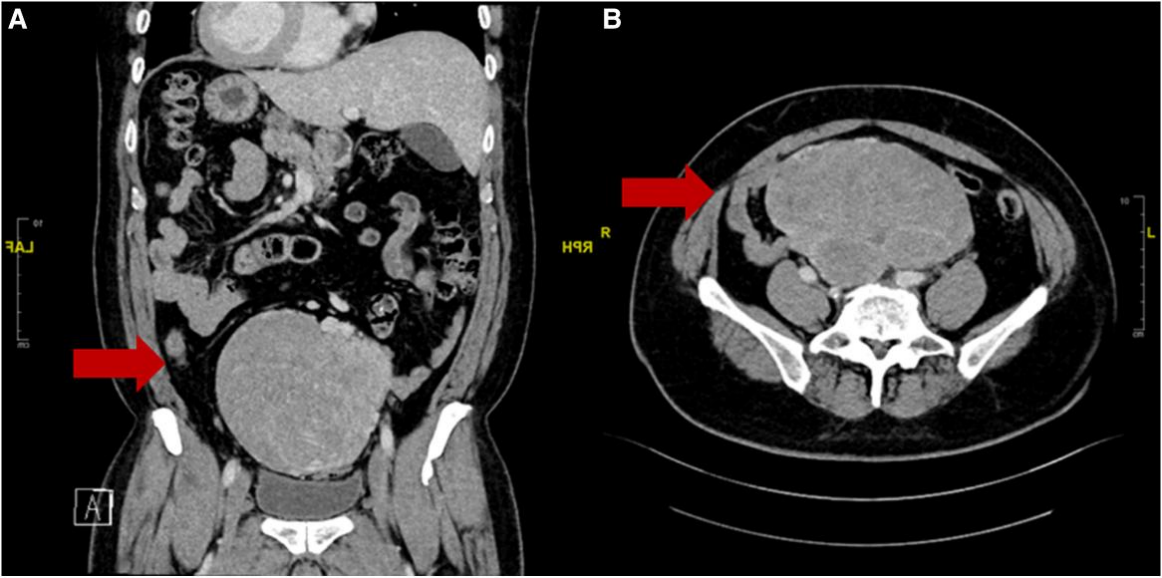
Computed tomography images of the abdomen and pelvis in the coronal (A) and transverse (B) planes demonstrating large heterogenous abdominal mass extending from suprapubic area to lower abdomen (arrows).
